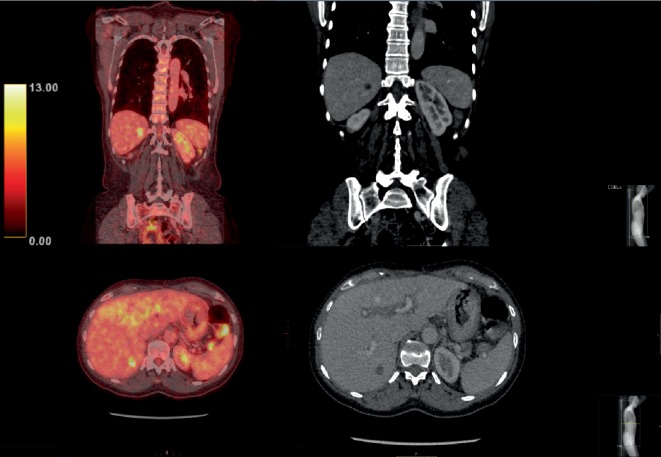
No change in the liver metastasis in segment 6 was observed upon evaluation of the response to first-line palliative chemotherapy. PET-CT (left column) and CT (right column) images. The SUVmax was 5.5 for the lesion and 3.1 for the surrounding liver.
